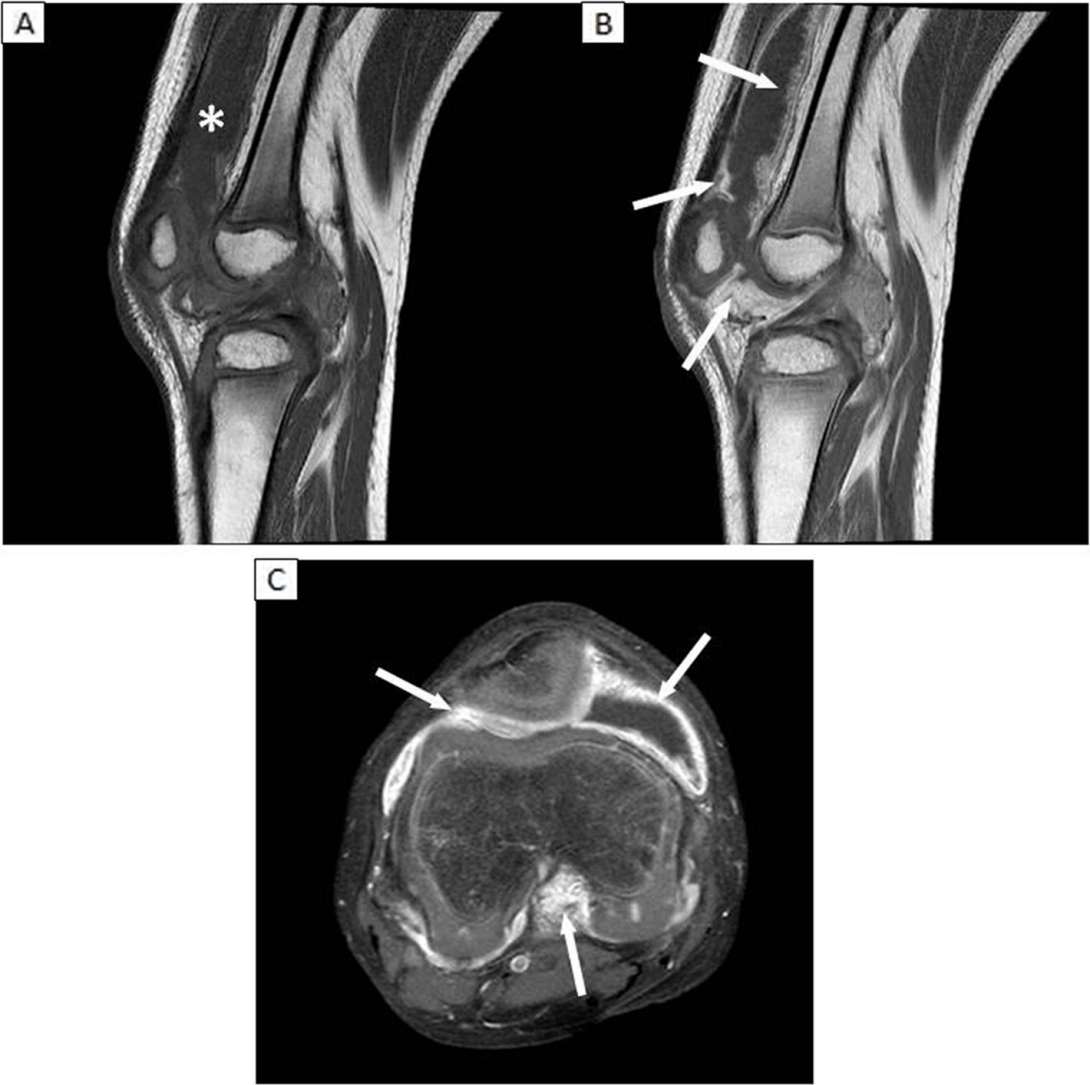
MR images of the right knee joint (obtained at age 8) revealing excessive joint fluid in the suprapatellar recess (see asterisk) and thickened enhancing synovial membrane throughout the entire joint (arrows). A Sagittal T1-weighted pre-contrast image; B Sagittal T1-weighted post-contrast image showing the thickened and enhancing synovial membrane in the suprapatellar, retropatellar and infrapatellar regions (arrows); C Axial T1-weighted fat-suppressed post-contrast image showing thickened enhancing synovial membrane in the retropatellar region and around the cruciate ligaments (arrows)
